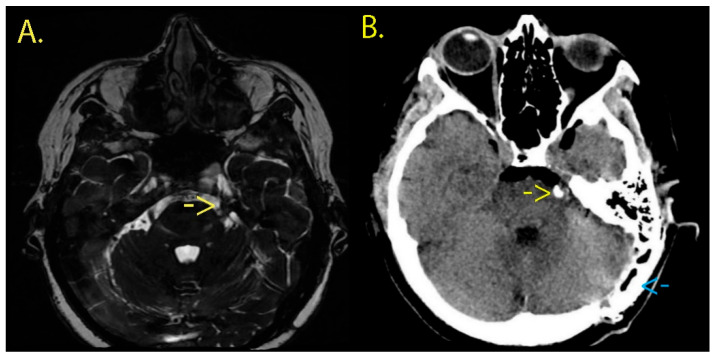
(A) Preoperative FIESTA-C sequence brain MRI revealing a neurovascular conflict on the left side (yellow arrow) between the left trigeminal nerve and left SCA. (B) Postoperative cerebral CT scan on the first day. The yellow arrow points out the new Teflon piece, while the blue arrow shows the cranioplasty cement used to cover the postoperative skull defect.
